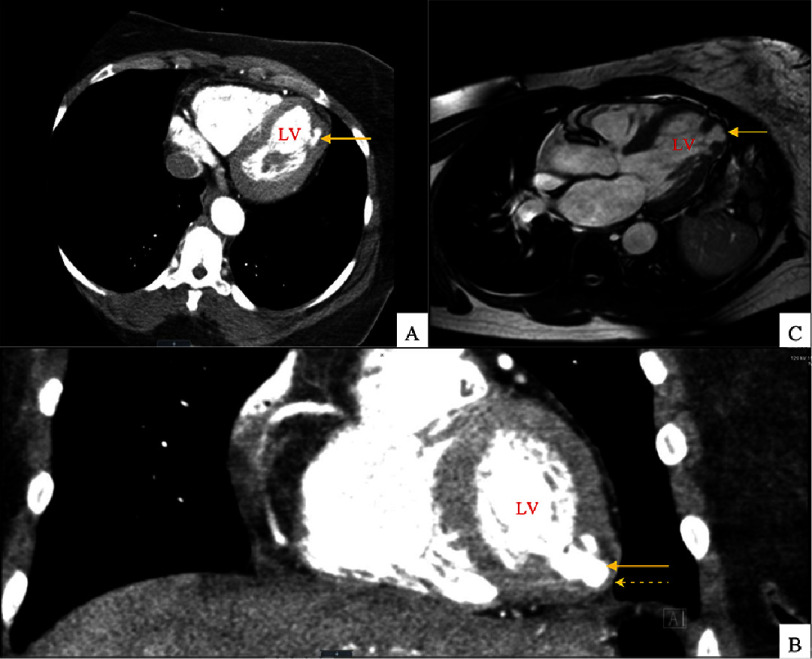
A and B show serial computed tomography (CT) images of a cleft-like deformity at the left ventricular apex (LV), giving rise to a prominent diverticular outpouching that extends laterally from the cleft (solid arrows). The diverticulum maintains continuity with the ventricular cavity through a narrow neck. Figure C shows a T2-weighted cardiac magnetic resonance imaging (MRI) of the outpouching represented as a focal, contractile diverticulum of the apical myocardium (solid arrow). The diverticular wall demonstrates localized myocardial thinning (broken arrow) yet preserves contractility relative to adjacent left ventricular segments.
